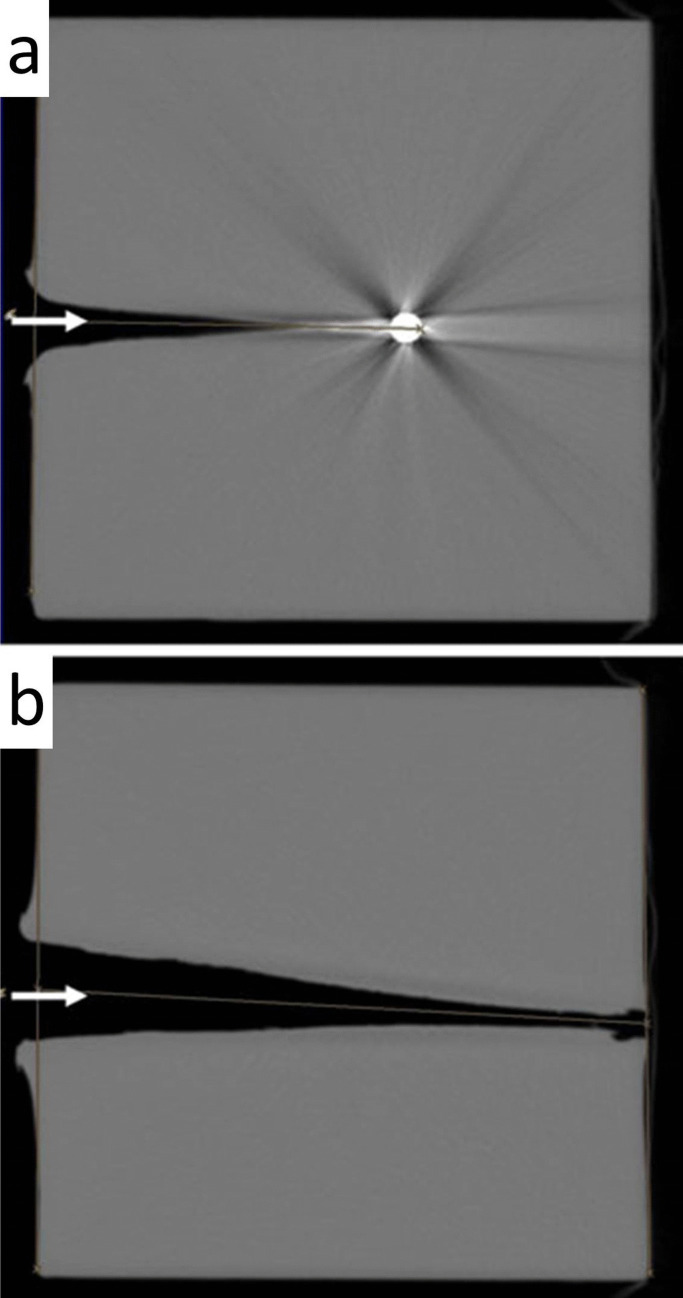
(cf. [50]) Contact shots into ballistic glycerin soap using different charges of black powder (1.5 and 2 g, respectively). The entrance sites are marked by arrows. The cavitations caused by the spherical lead balls are visualized by computed tomography. Note the conical shape of the cavitations. In the upper image (a), the bullet got stuck after a distance of 15 cm (1.5-g charge). In the lower image (b), the projectile travelled through the whole thickness of the block (25 cm)
